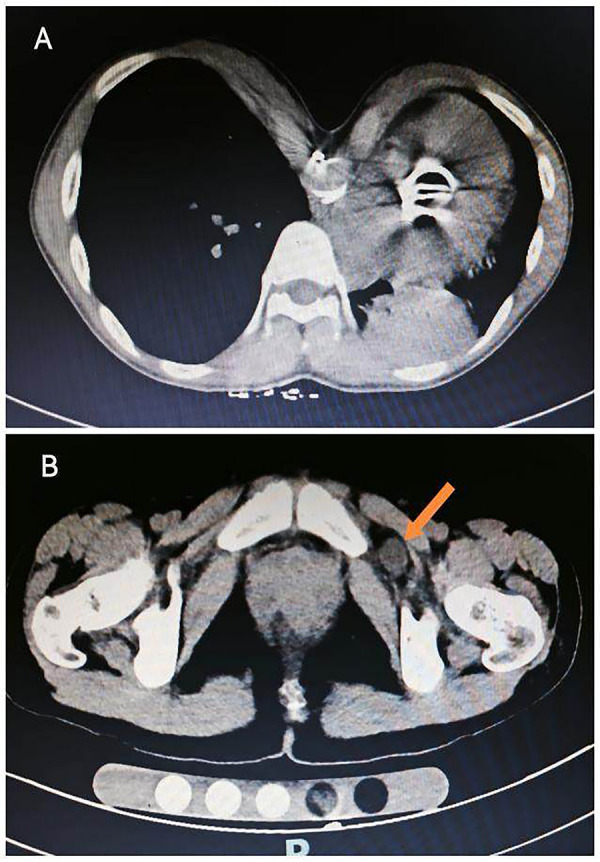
(A) chest CT showing cardiomegaly, post aortic valve replacement, pectus excavatum; (B) pelvic CT showing a left obturator hernia (the hernial contents between the external obturator muscle and the pectineus muscle are indicated by the orange arrow).
